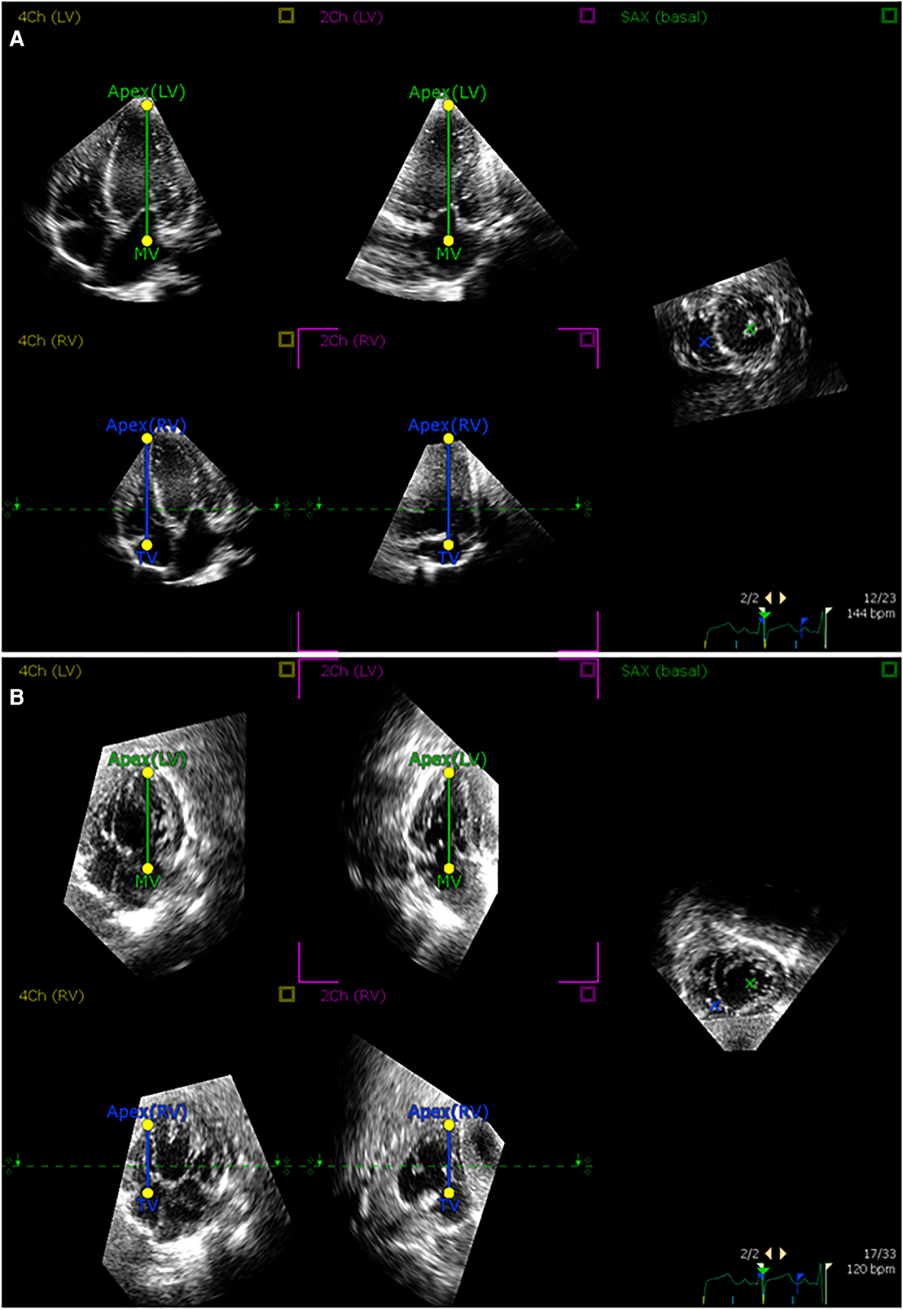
Alignment of 3D echocardiographic images from apical and subcostal views. An example of alignment of (A) apical and (B) subcostal views in the postprocessing software according to prespecified landmarks.
